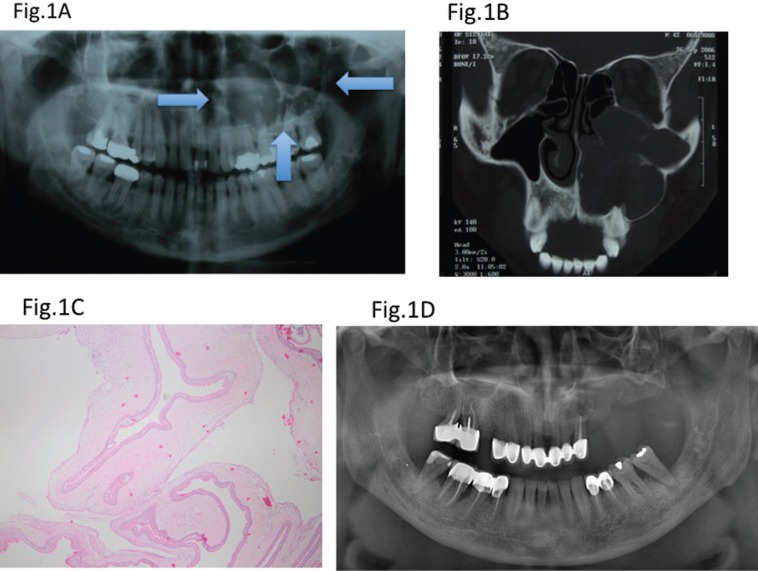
A) Multilocular radiolucent lesion in the posterior left maxillary region; B) Computed tomography (CT) images revealed that lesion had expanded the floor of the orbita and collapsed the left nasal passage; C) Thin, parakeratotic epithelial lining covering the cyst cavity was observed. The fibrous wall is devoid of inflammatory cells, and a small daughter cyst is seen within the fibrous wall. D) A panoramic radiography control after 8 years.
